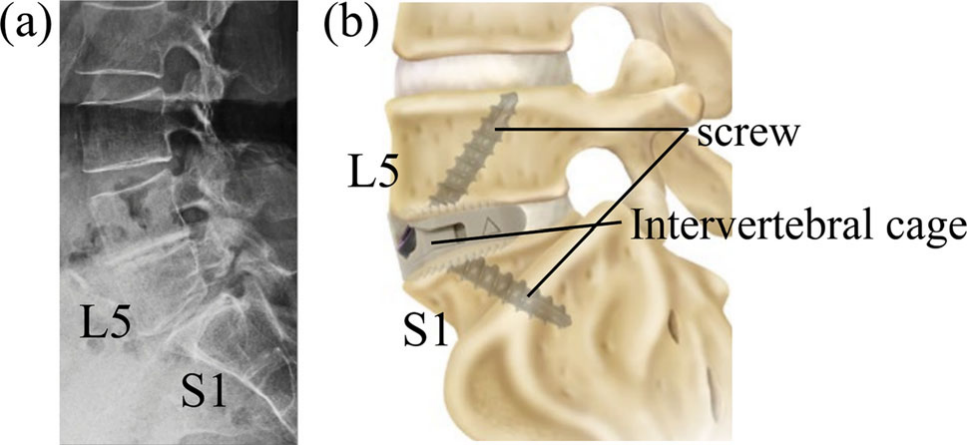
a Representative X-ray image of spondylolisthesis: L: lumbar, S: sacrum. b Post-operative image of OLIF51 using intervertebral Cage fixed with vertebral fixation screws
